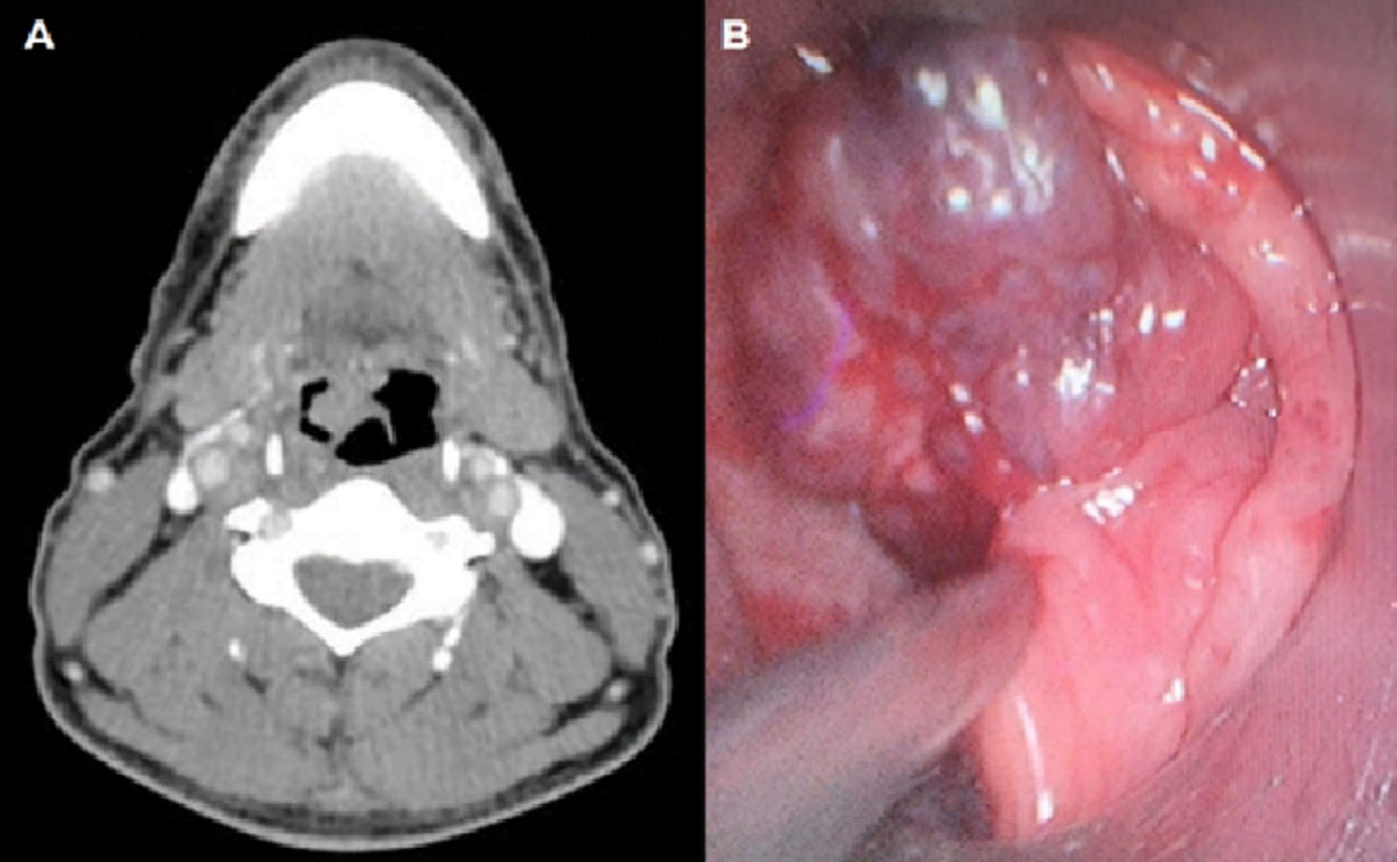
Computed tomography (A) and direct laryngoscopy (B) at the time of incisional biopsy, demonstrating a vallecular lesion suggestive of hemangioma.
